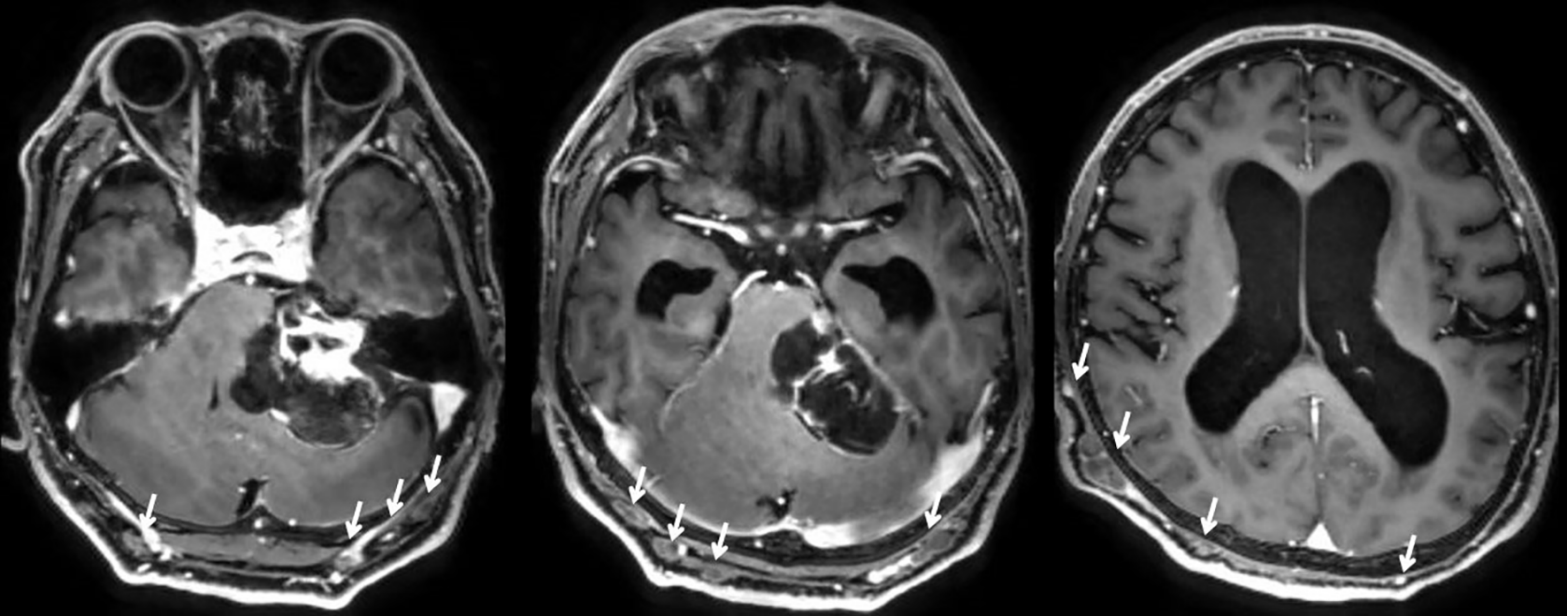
Preoperative axial contrast enhanced T1-weighted MRI demonstrating a mass lesion in the left cerebellopontine angle. The lesion shows well-enhanced solid components and cystic components, causing significant compression of the cerebellum and brain stem, along with associated hydrocephalus. Additionally, multiple subcutaneous mass lesions are observed (white arrows).
